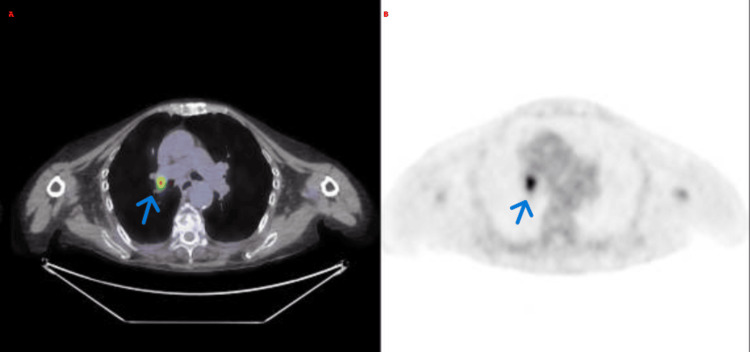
PET/CT of the whole body (A–B) Fused PET/CT and PET-only axial images showing a hypermetabolic lesion in the right supra-hilar pulmonary. PET/CT: Positron emission tomography/computed tomography.
